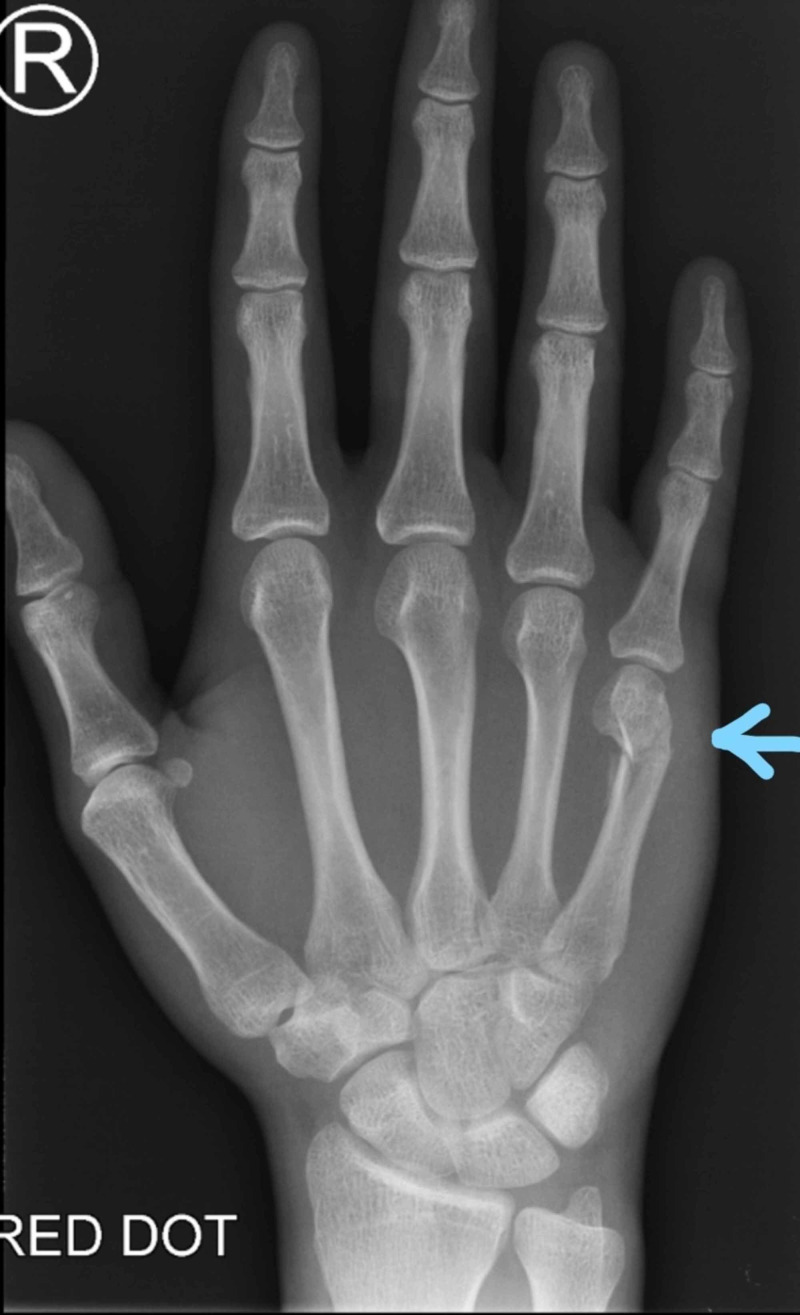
X-ray (PA view) of the right hand shows an extra-articular, comminuted fracture of the neck of the fifth metacarpal PA = Posteroanterior
